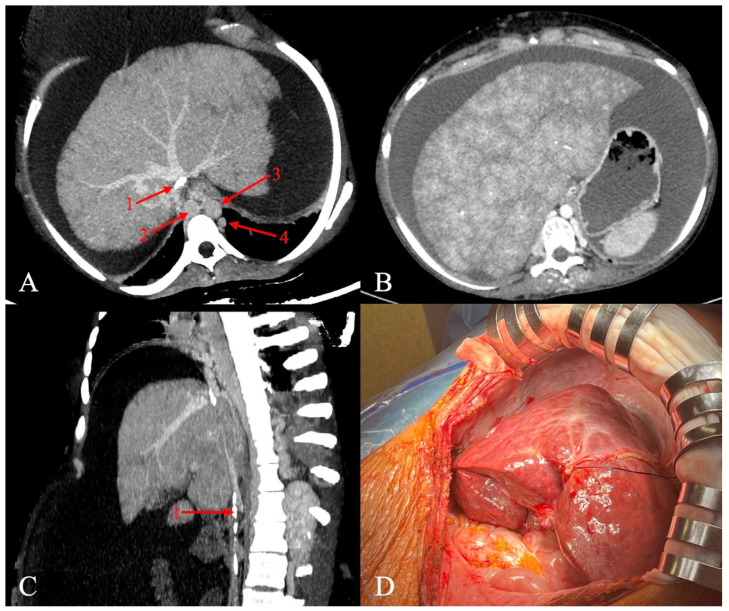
Pretransplant CT scans (A–C) and intraoperative picture of the recipient’s native liver (D). The pretransplant recipient CT scans show calcified IVC (red arrow 1; (A,C)) and dilated azygos (red arrow 2, (A)) and hemiazygos veins (red arrow 4, (A)), maintaining blood flow from the lower part of the body. The CT scan (nutmeg liver, (B)) and intraoperative picture (D) show perfusion abnormalities on account of chronic venous congestion. CT, computed tomography; IVC, inferior vena cava; red arrow 3, aorta.
